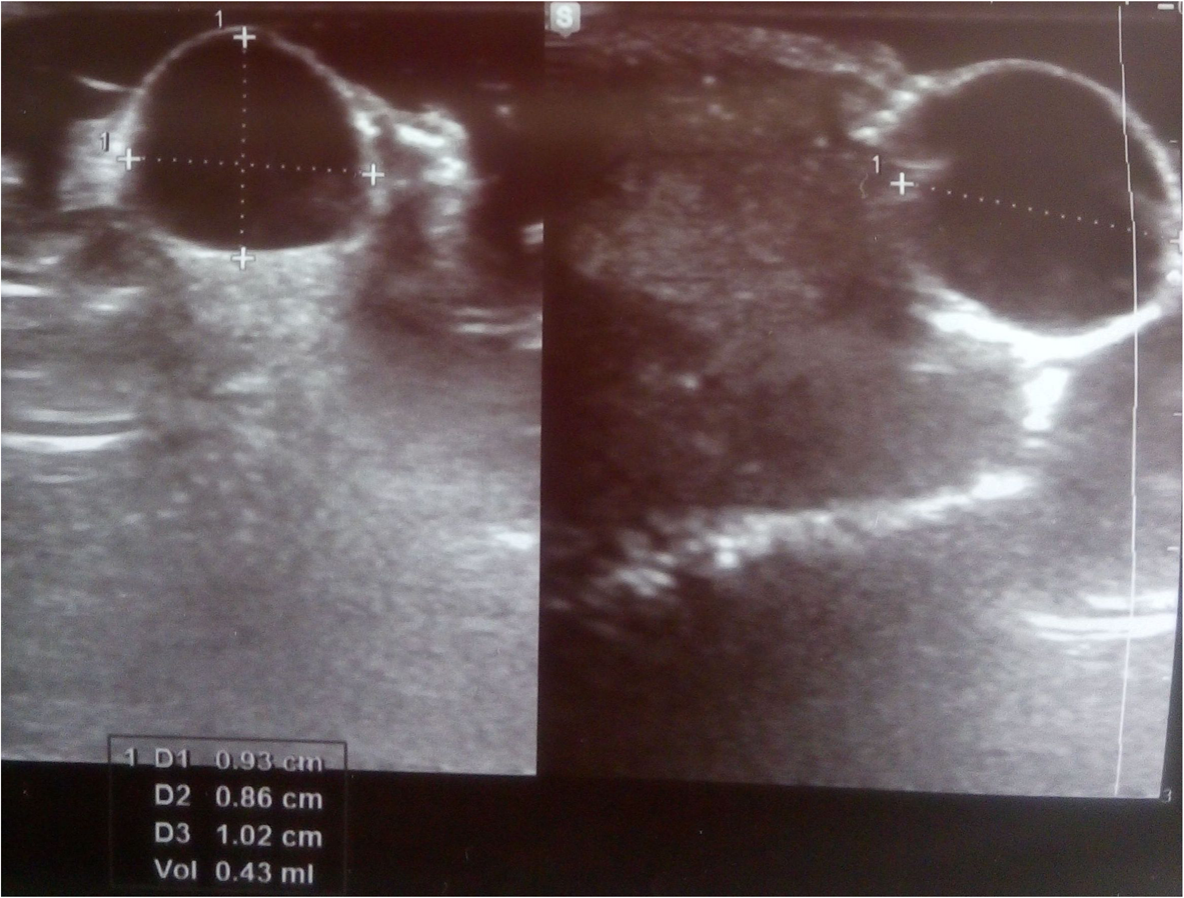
Ultrasonography of the cyst showing a well-circumscribed isoechoic lesion without any extension to the urethra
